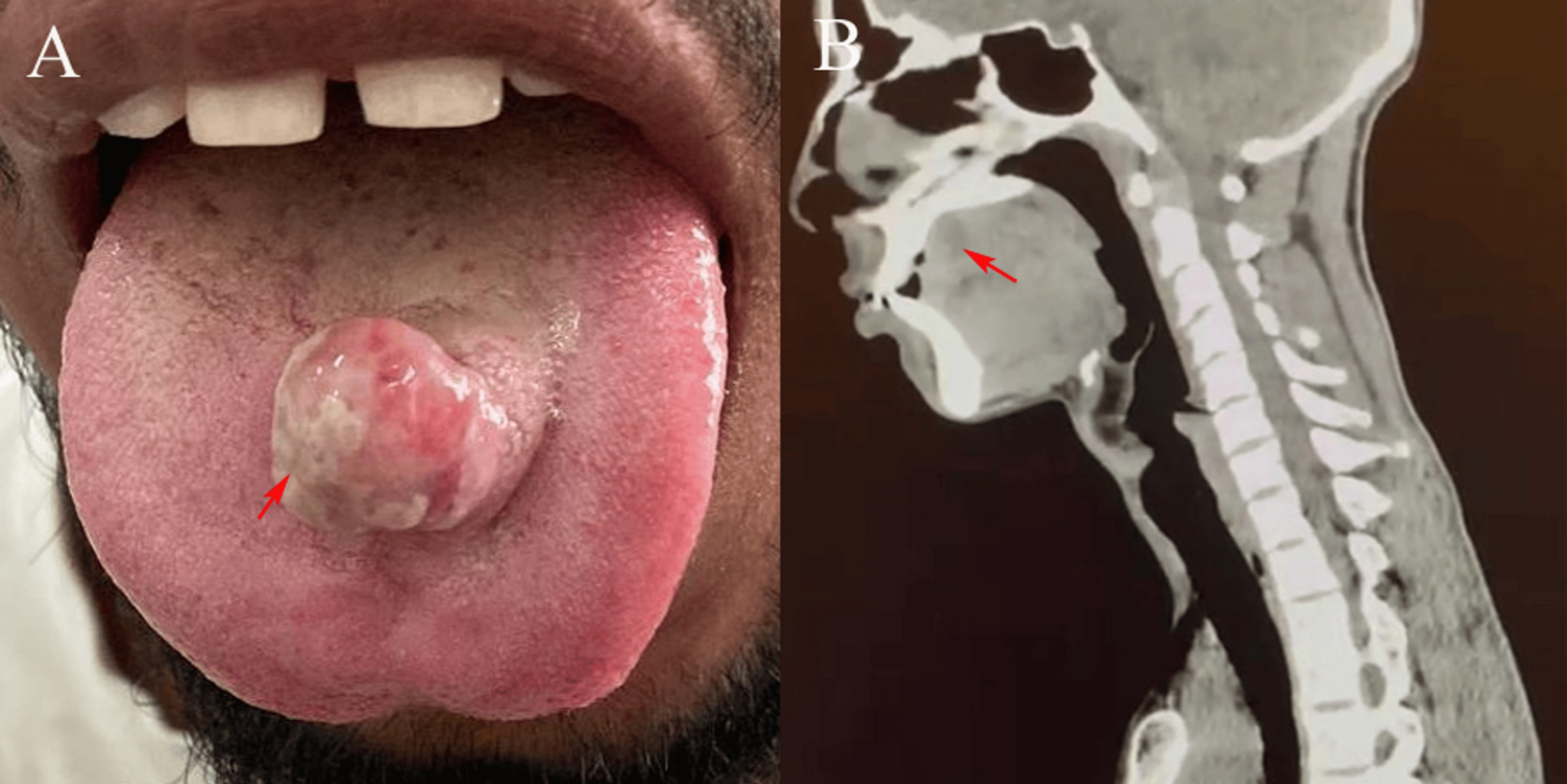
Clinical and saggital CT images of the patient. (A) Clinical photograph showing a lobulated, firm, well-circumscribed 4–5 cm mass on the mid-dorsal surface of the anterior two-thirds of the tongue (arrow). (B) Sagittal non-contrast computed tomography of the neck demonstrating a well-defined hypodense lesion on the tongue dorsum (arrow) with no invasion into adjacent musculature or deeper structures.
